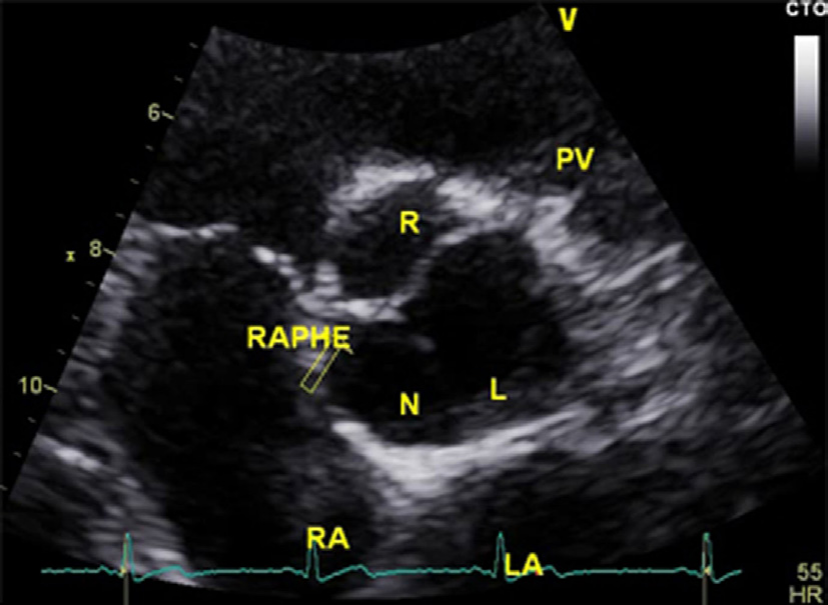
Follow-up transthoracic echocardiography performed 5 months after presentation, showing the presence of previously undiagnosed bicuspid aortic valve.
